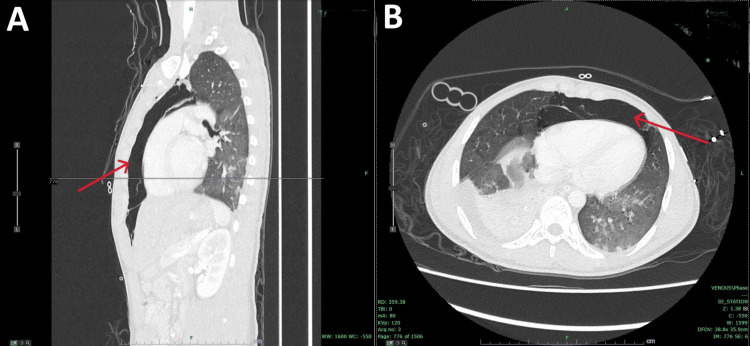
Computed tomography of the chest (A) Sagittal CT view showing pneumomediastinum (red arrow). (B) Axial CT view demonstrating pneumomediastinum (red arrow).
